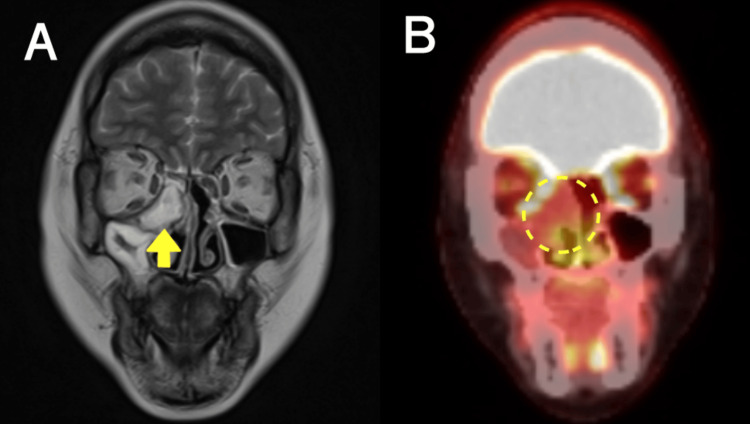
MRI and PET/CT images obtained three months after proton beam therapy A. Coronal T2 MRI showing complete remission with necrotic change (arrow). B. PET/CT demonstrating reduced activity of the mass (dashed line). MRI, magnetic resonance imaging; PET/CT, positron emission tomography/computed tomography
